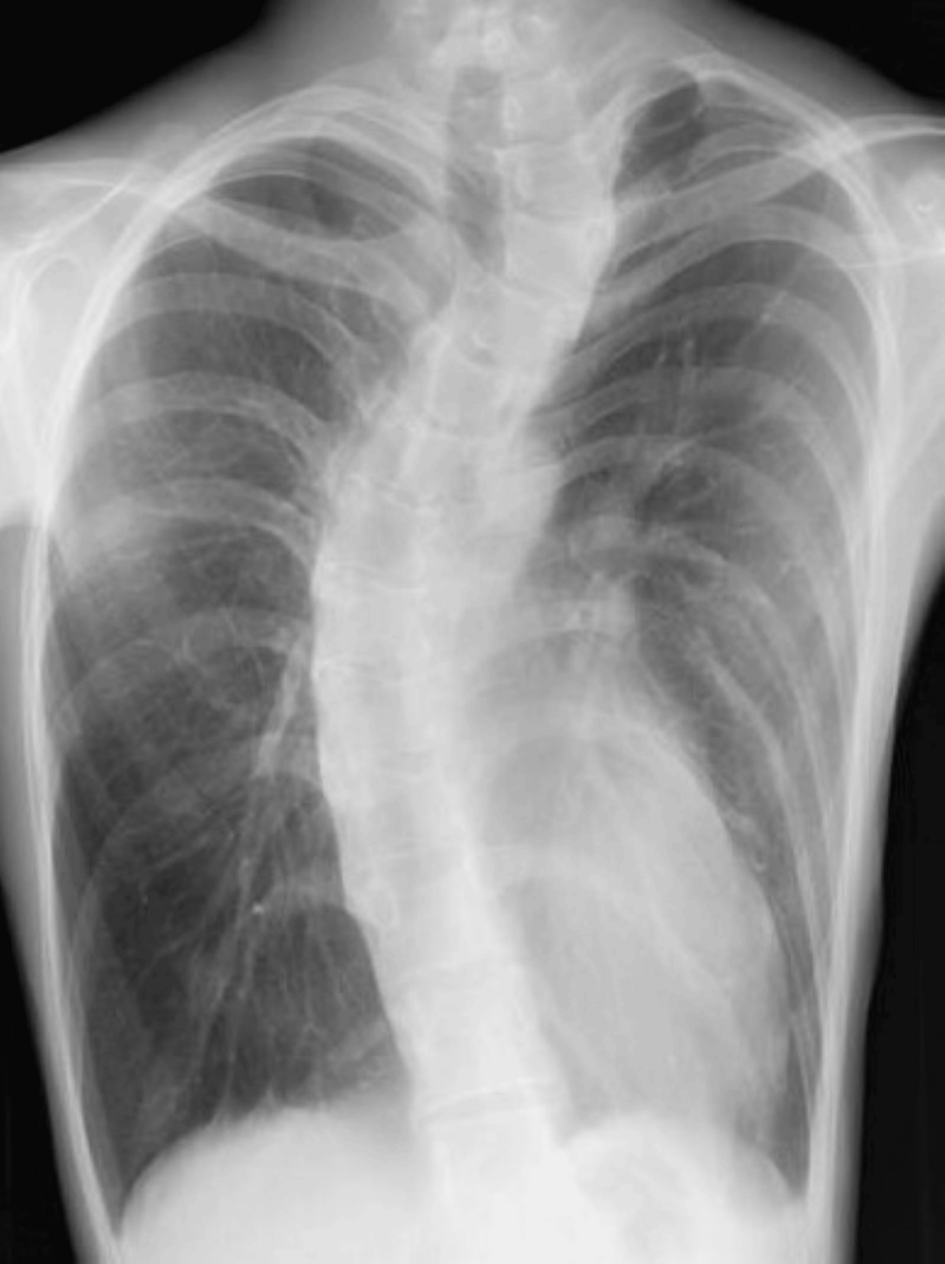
Postoperative chest radiograph. Full expansion of the right lung was obtained.
